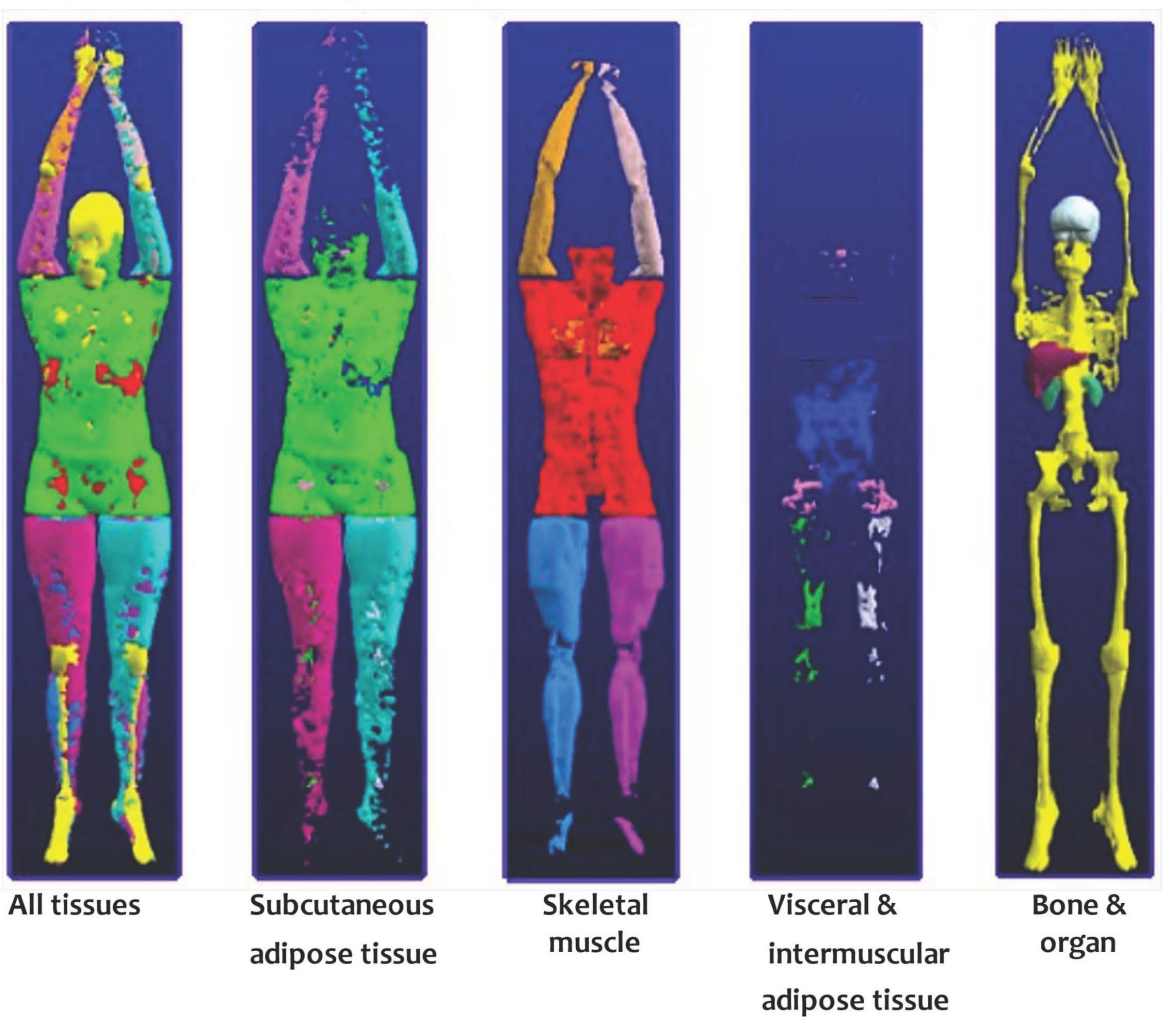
Whole‐body magnetic resonance imaging—shown are three‐dimensional reconstructions of segmented tissue and organ compartments. Image adapted from Shen et al. with permission [106].
